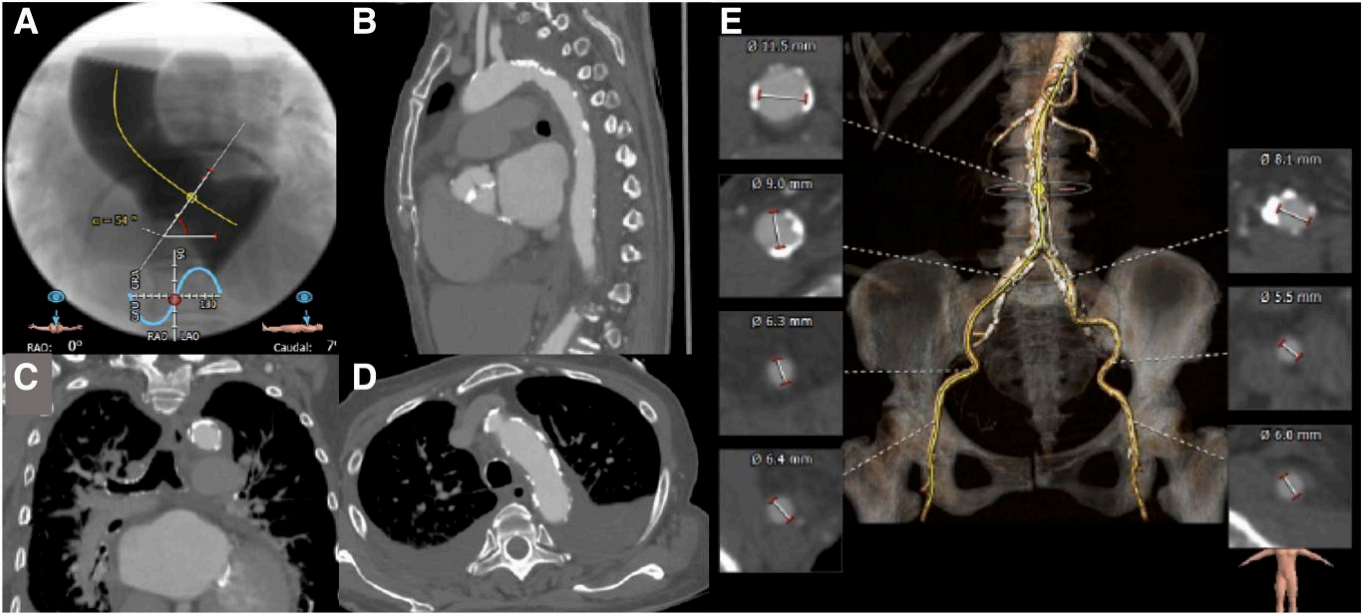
Computed tomography angiography images. (*A*) Aortic root angle. (*B*) Sagittal view of the aortic arch. (*C*) Coronal view of the aortic arch. (*D*) Axial view of the aortic arch. (*E*) Iliofemoral access route.
